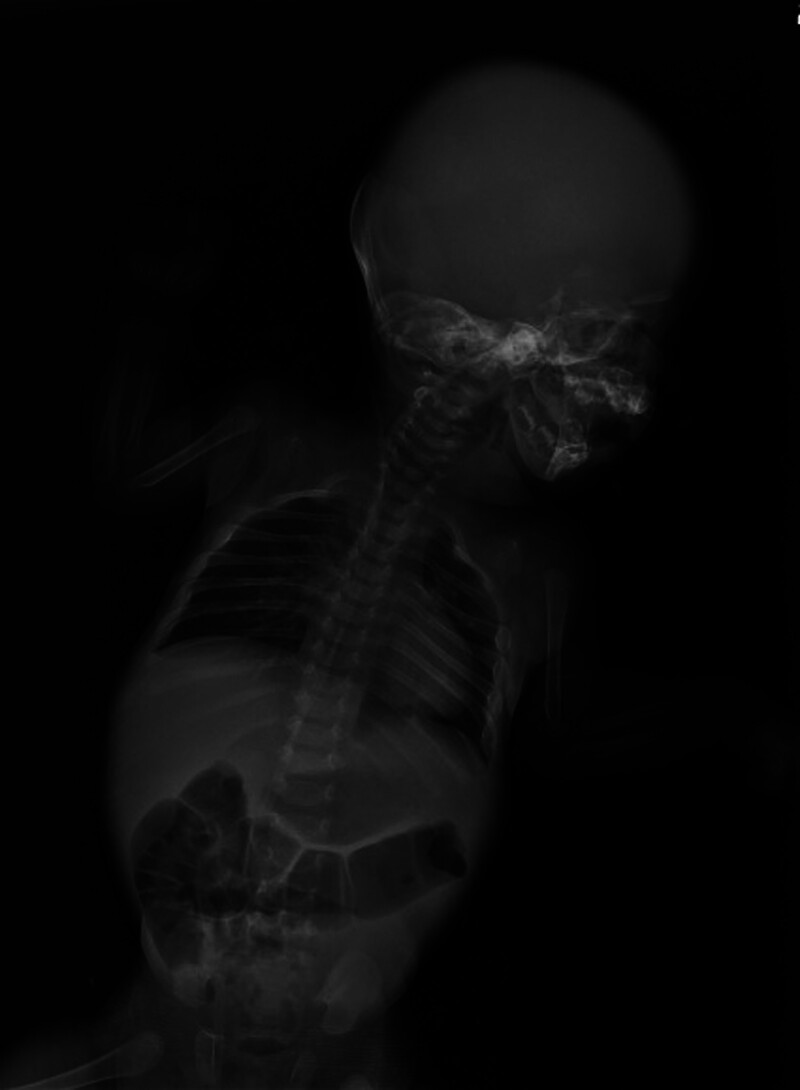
Head X-ray showed the absence of frontal, temporal, and bilateral parietal bones.
